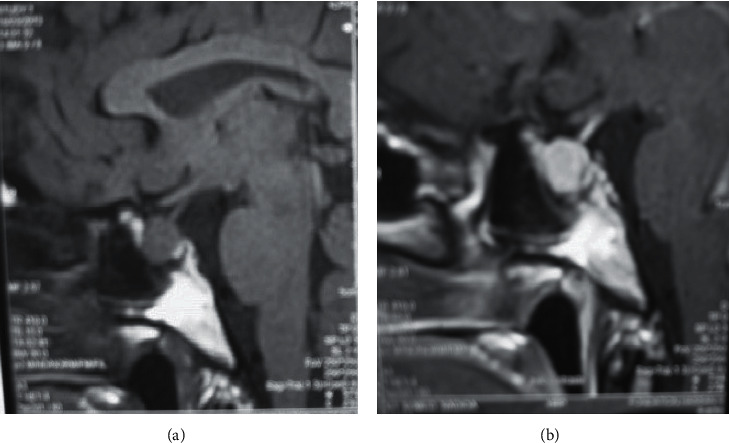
Preoperative pituitary MR images (T1-weighted, contrast, and sagittal view) showing (a) pituitary macroadenoma 12 mm without mass effect on adjacent structures (especially optic chiasm) and (b) strong homogeneous enhancement of the pituitary adenoma after contrast administration.
